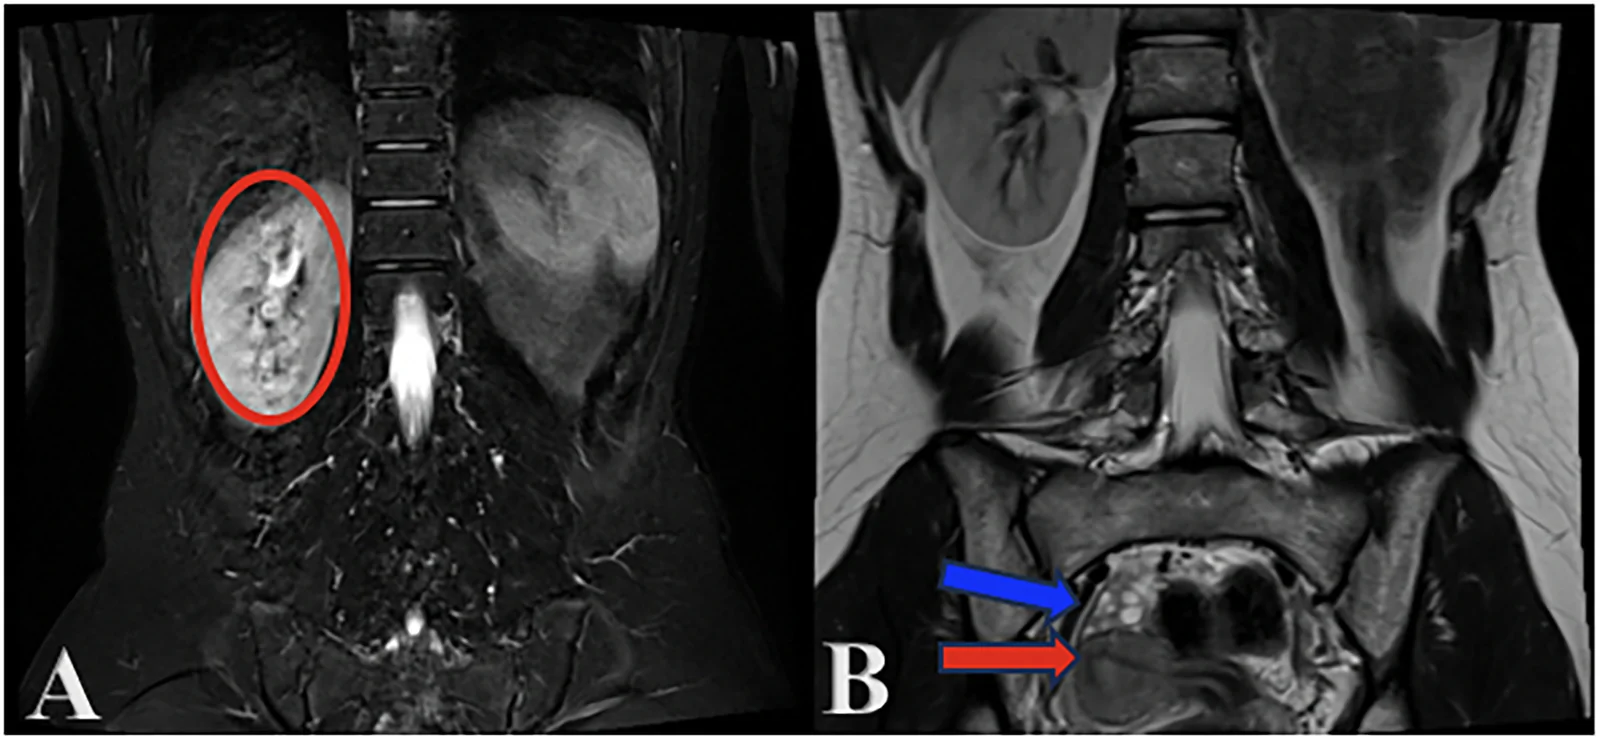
Abdominal MRI demonstrating renal anomaly associated with OHVIRA syndrome. (A) Coronal T2-weighted STIR image demonstrating a normal right kidney (marked with a red circle), with absence of the left kidney, consistent with left renal agenesis. (B) Coronal T2-weighted image showing the normal right kidney, absence of the left kidney, and visualization of the normal right uterus (marked with a red arrow) and ovary (marked with a blue arrow).
